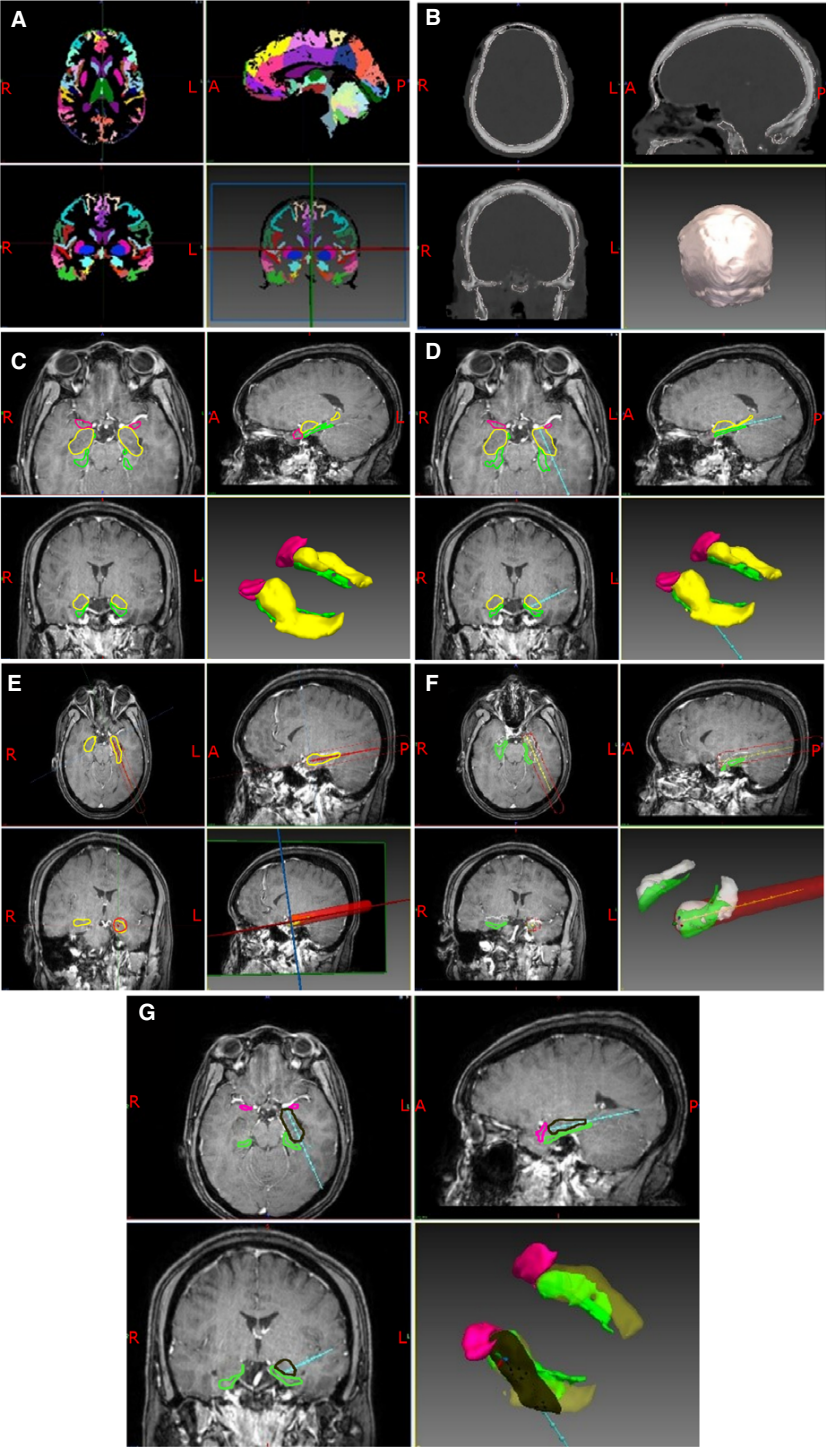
A, T1‐weighted MRI scans for each patient were used to generate geodesic information flow (GIF) brain parcellations. The whole brain is segmented into 140 separate anatomical structures that can be used to guide trajectory planning and model generation. B, Pseudo‐CT images were generated from the same T1‐weighted MRI scans to provide an image from which a model of the skull can be extracted. The external surface of the skull model is used to calculate the trajectory drilling angle, and the inner surface is used to calculate intracranial trajectory length. C, Models of the cortex, lateral ventricle, amygdala, hippocampus, entorhinal cortex, parahippocampal gyrus, gray matter ribbon, inferior occipital gyrus, middle occipital gyrus, inferior temporal gyrus, middle temporal gyrus, intracranial mask, and sulci are extracted from the GIF parcellation and combined with the skull model. In the image shown, the amygdalohippocampal complex is colored in yellow, entorhinal cortex in pink, and parahippocampal gyrus in green. The remaining models have been excluded for clarity. D, Based on the generated models the optimal trajectory is calculated to target the amygdala while preventing entry to the lateral ventricle, thereby maximizing contact with the hippocampus, distance from sulci and vasculature, and minimizing intracranial trajectory length and drilling angle to the skull. The calculated laser trajectory is shown in blue. E, A region of ablation is then modeled along the model laser trajectory. The Visualase system can ablate a diameter between 5 and 20 mm. A conservative maximum ablation diameter of 15 mm was applied to the model (red cylinder). F, Areas of overlap between the modeled laser ablation zone and the anatomical regions of interest were then extracted so that an estimation of the modeled ablation cavity could be calculated. The volume of each of the regions of interest within the modeled ablation cavity were calculated individually and as a whole. Amygdalohippocampal complex is shown in white and parahippocampal gyrus in green. G, Expected ablation cavity within the ROIs (black) showing the extent of mesial hippocampal head ablation. Amygdalohippocampal complex is shown in yellow, parahippocampal gyrus in green, and entorhinal cortex in pink
